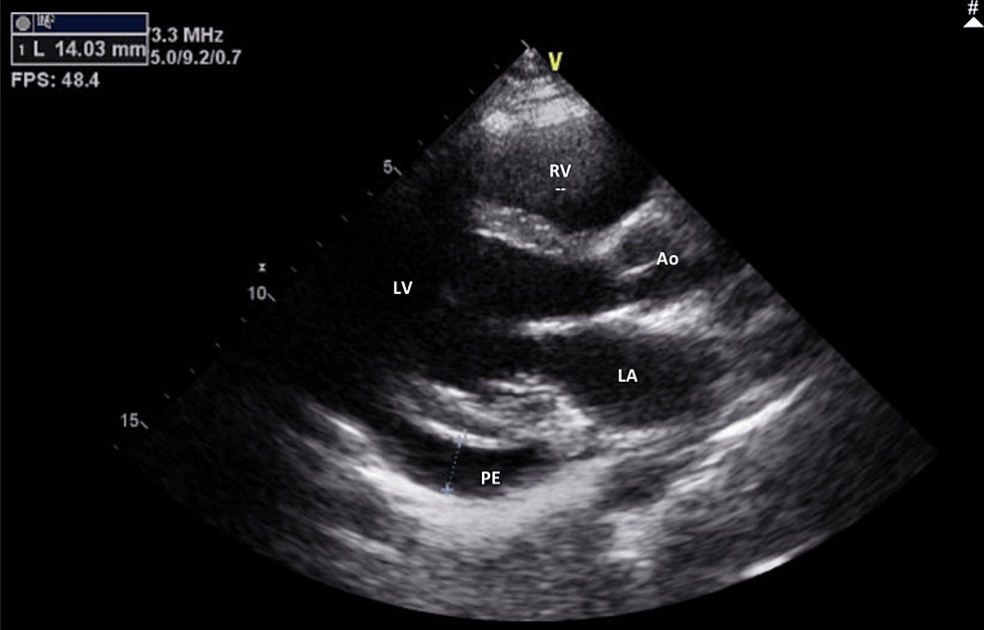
Echocardiogram (parasternal long-axis view) after pericardiocentesis demonstrating residual pericardial effusion of moderate volume PE: pericardial effusion; LA: left atrium; LV: left ventricle; Ao: aortic valve; RV: right ventricle
